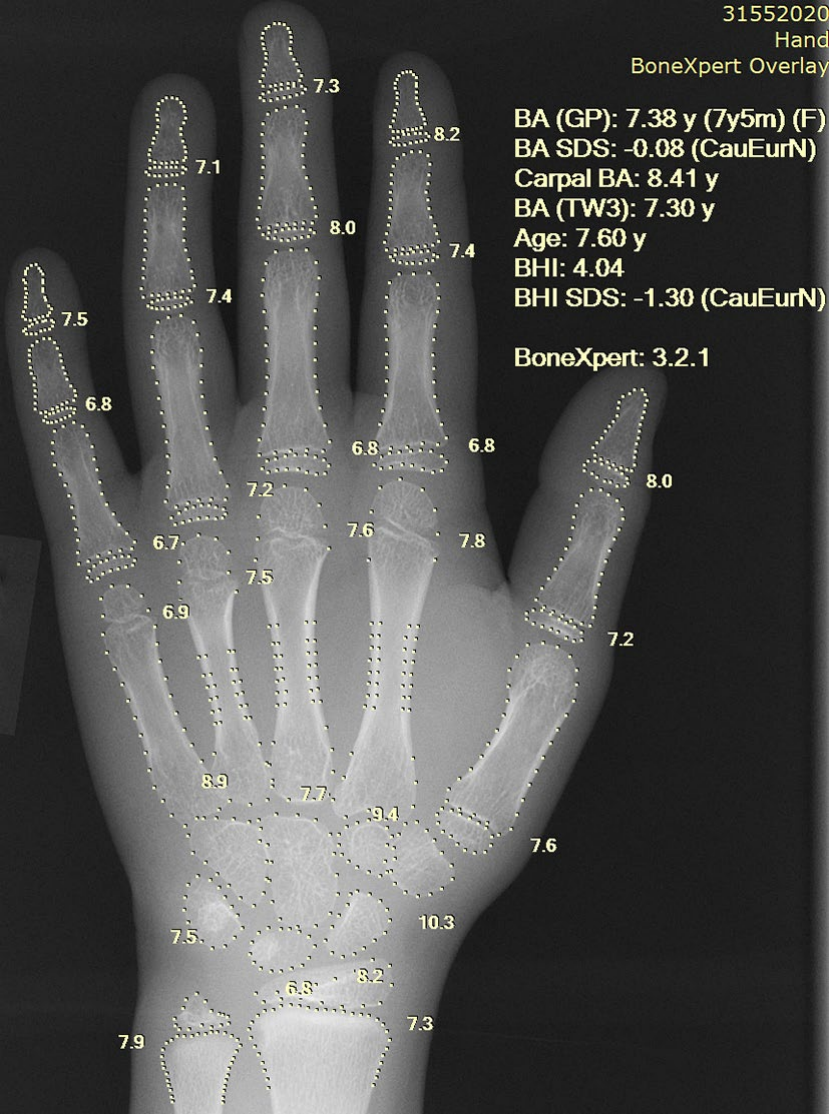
Posterior-anterior left-hand radiograph with bone age assessment by BoneXpert. This is the result file automatically placed in the same study in the digital archive. BA = bone age, averaged over the 21 tubular bones; BHI = Bone Health Index; SDS = standard deviation score; GP = Greulich-Pyle; TW3 = Tanner-Whitehouse Version 3.
